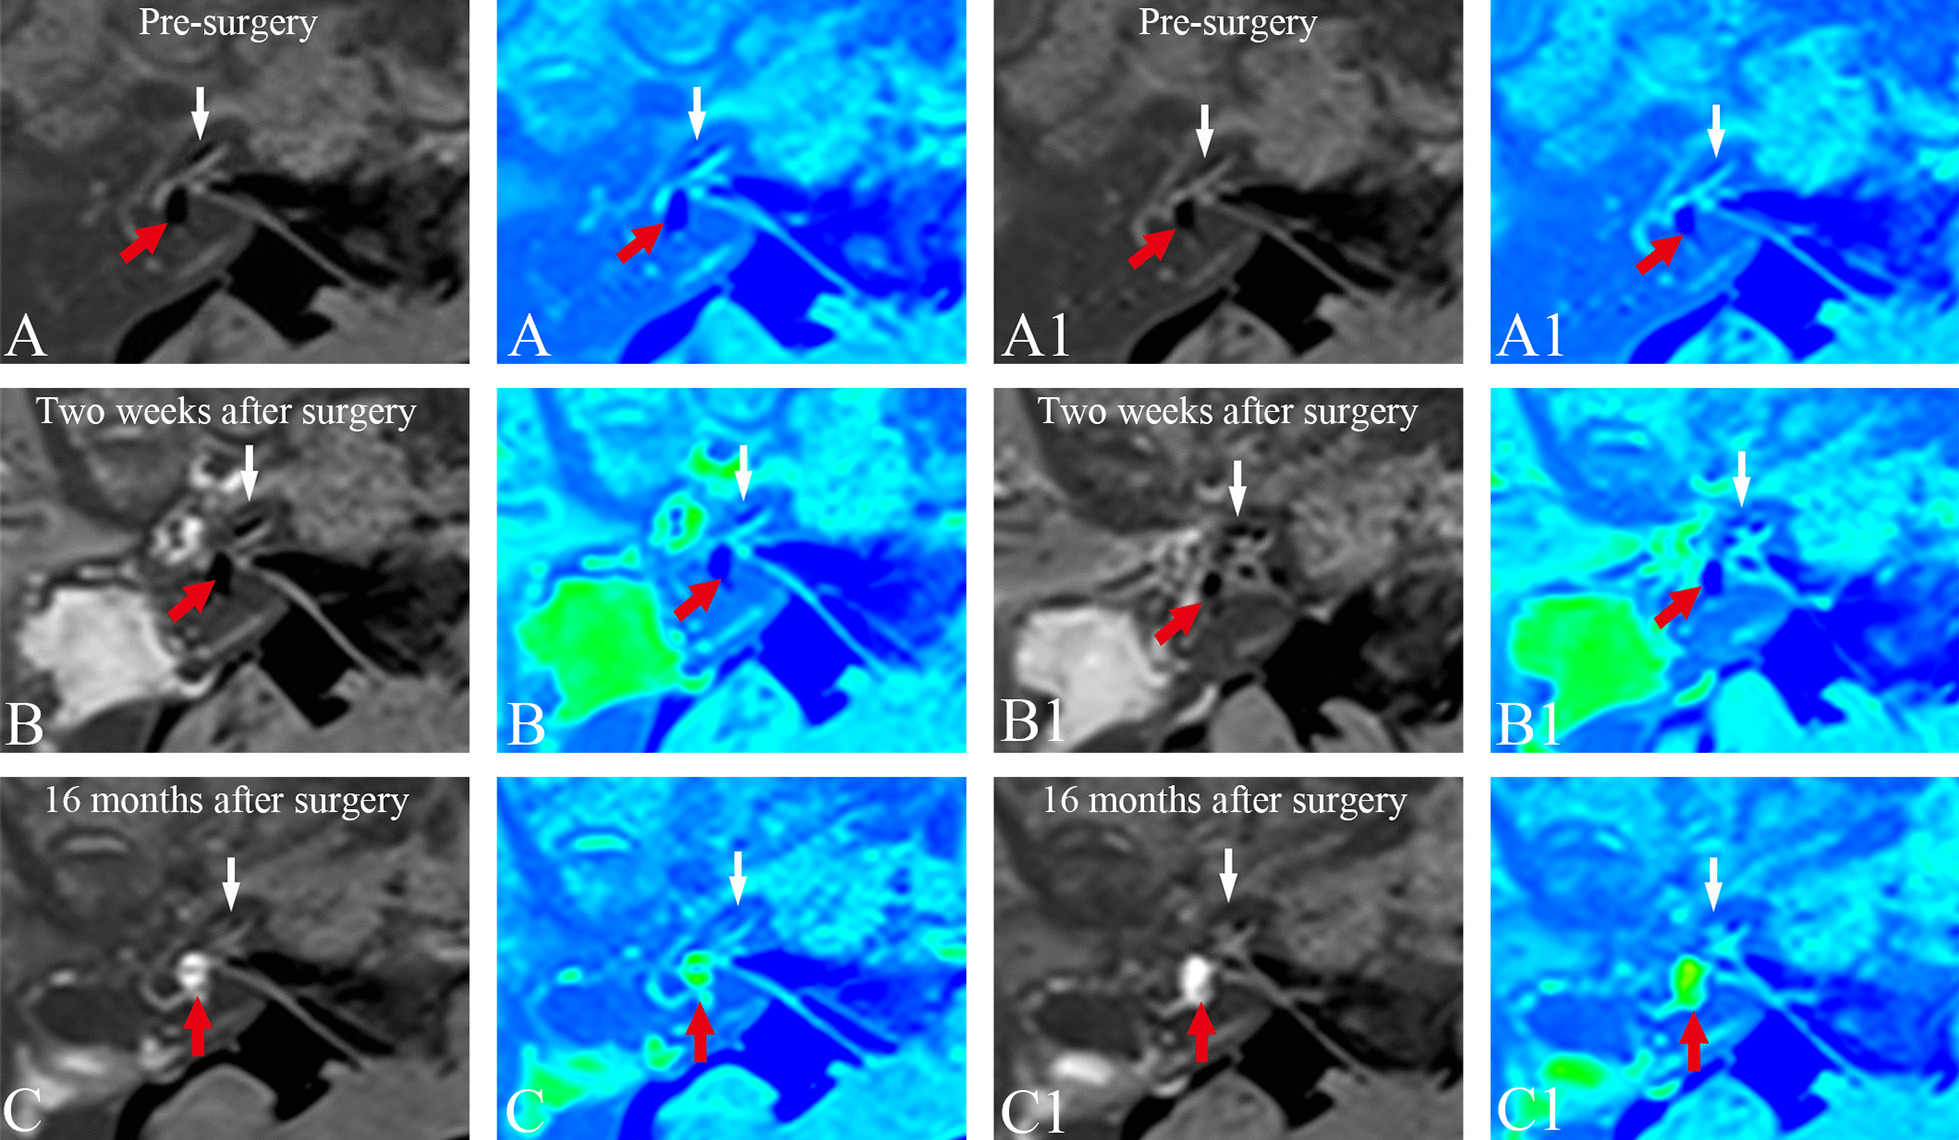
Serial MRI axial scans (left column) and the corresponding MRI colored images (right column) revealed a significant EH both in the cochlea (white arrow) and vestibule (red arrow) prior to surgery (A, A1), a tendency for EH to temporarily increase both in the cochlea (white arrow) and the vestibule (red arrow) 2 weeks after surgery (B, B1) and a complete reversal of vestibular hydrops (red arrow) and downgrading of cochlear hydrops (white arrow) from grade II to grade I 16 months after surgery (C, C1) in the same patient in Fig. 2. *EH* endolymphatic hydrops, *MRI* magnetic resonance imaging
